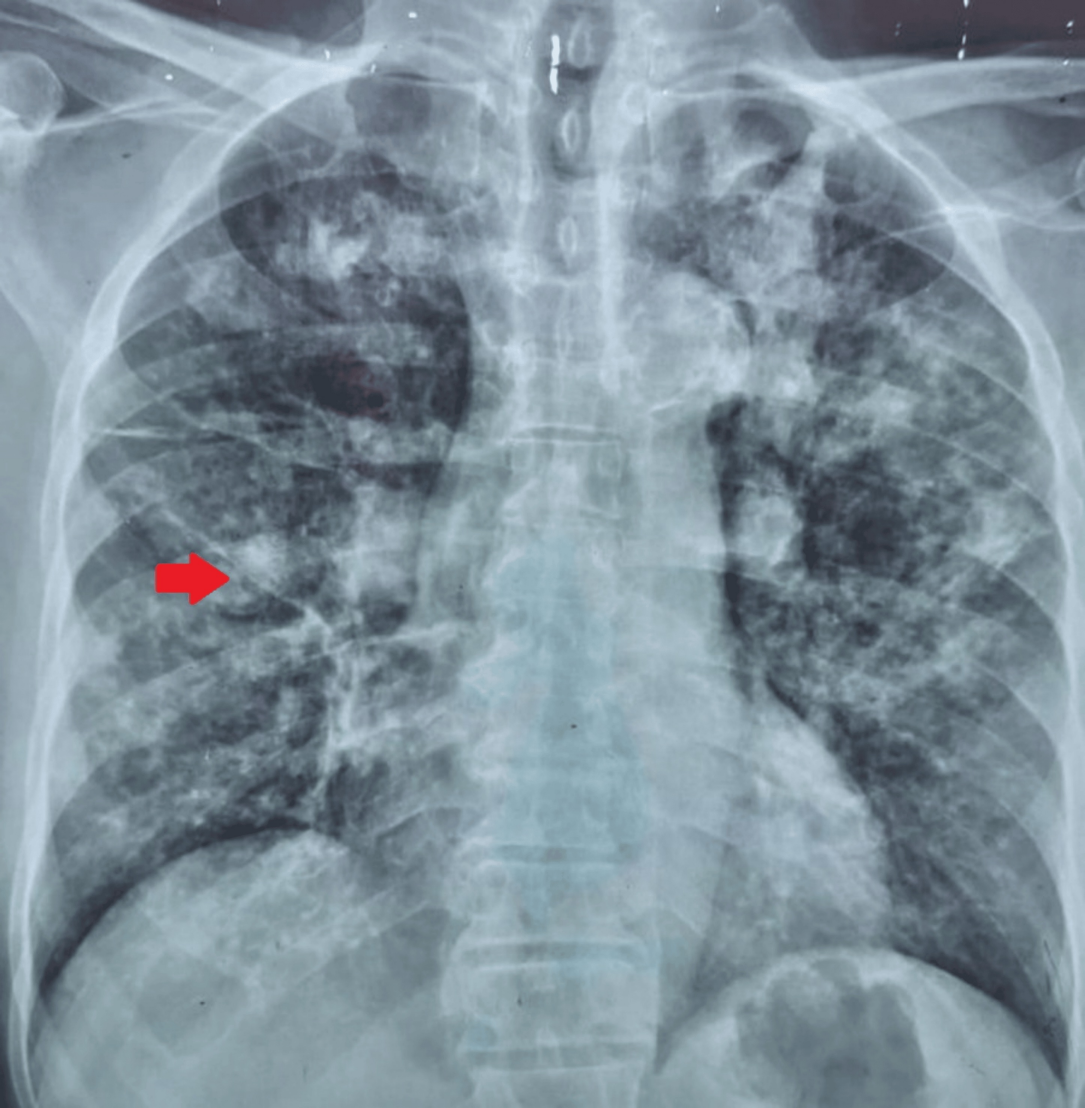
Chest radiograph (patient 1) Chest radiograph showing bilateral patchy consolidations with nodularity. The red arrow indicates a nodule in the right middle lobe close to the right hilum
